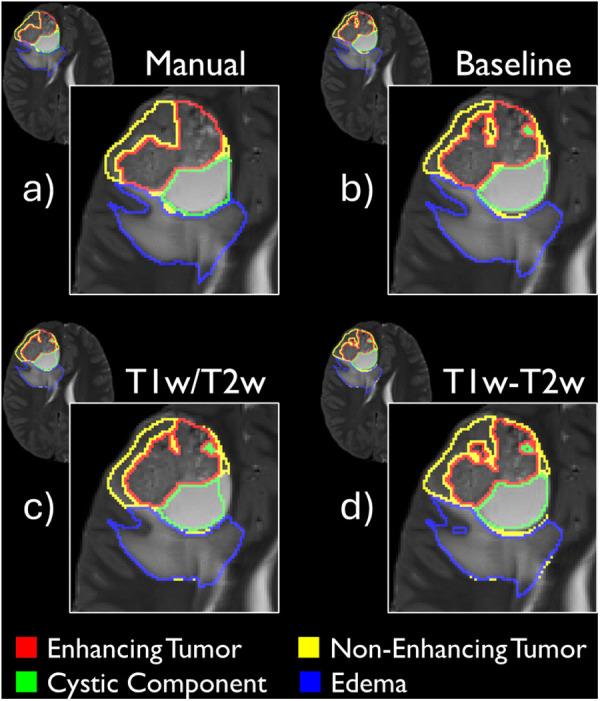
Example segmentation for a single case. Example segmentation executed by a) manual segmentation, b) the baseline model, c) the T1w/T2w ratio map and d) the T1w-T2w combined map. Segmentations are overlaid on the T2w MRI for visualization purposes. Example case was selected as the case with the individual Dice score for the whole tumor (ET + NET + CC + ED) which was closest to the median Dice score, whilst also having each tumor subregion present (e.g., not a case without ED for instance).
